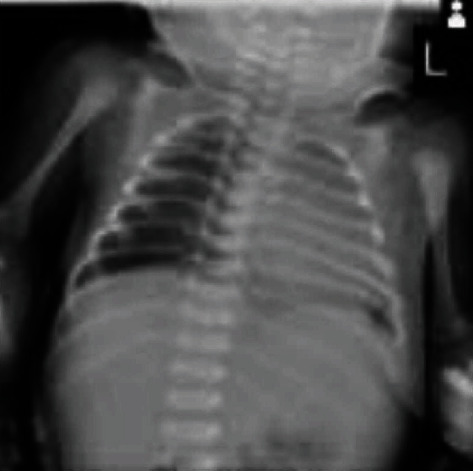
Chest radiograph of the newborn with diagnosis of transient tachypnea of the newborn (TTN).
